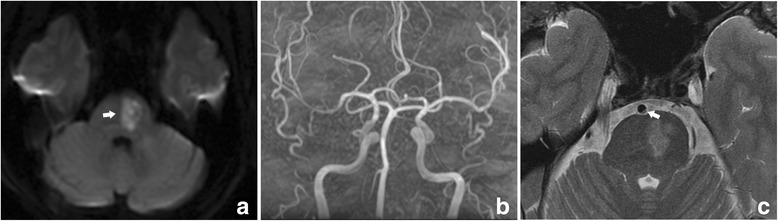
In a patient (50-59 year old) with acute isolated pontine infarction, (a) DWI showed a paramedian infarct in the left pons (arrow); (b) MRA showed no obvious stenosis in the basilar artery; (c) T2 dark fluid SPAIR showed a plaque relevant to the infarct (arrow)
